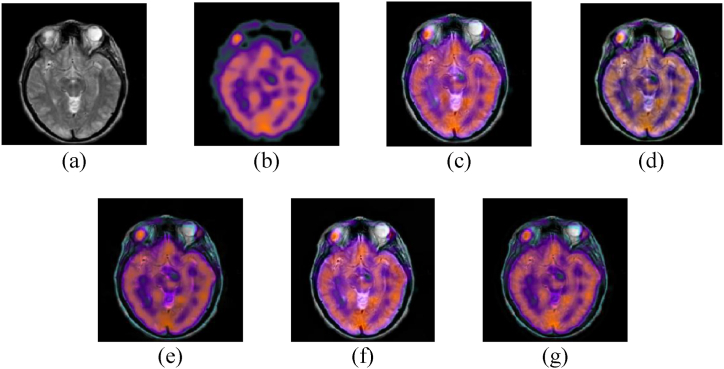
MRI and SPET images fusion results of Hypertensive encephalopathy disease, (a) MRI image, (b) PET image, (c) NSST-PAPCNN [24], (d) CNN [25], (e) CSMCA [26], (f) IFCNN [27], (g) Proposed.
